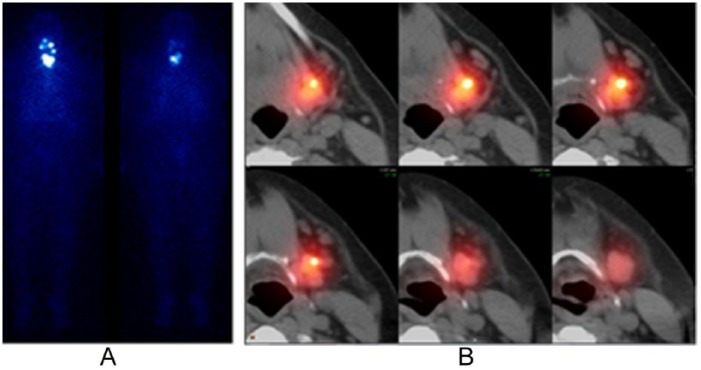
Parotid duct stone resulting in obstruction and inflammation as seen on WBS and SPECT/CT. A 50-year-old woman with a history of PTC was treated with surgery and 100 mCi of I-131. Posttherapy WBS (3A) demonstrates left-neck activity that on SPECT/CT (3B) corresponded to a stone impacted in the submandibular salivary gland duct. Abbreviations: WBS, whole body scan; SPECT/CT, single-photon emission computed tomography.
